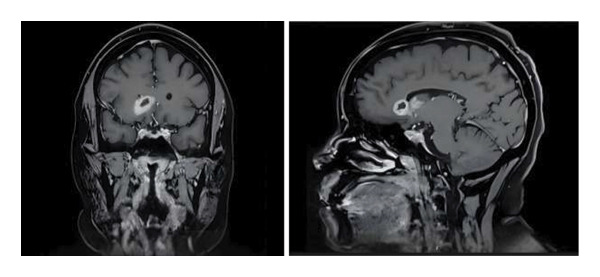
Repeat T2 FLAIR brain MRI with and without contrast—showing worsening rim—enhancing lesions within the right anterior frontal lobe/corpus callosum genu.
